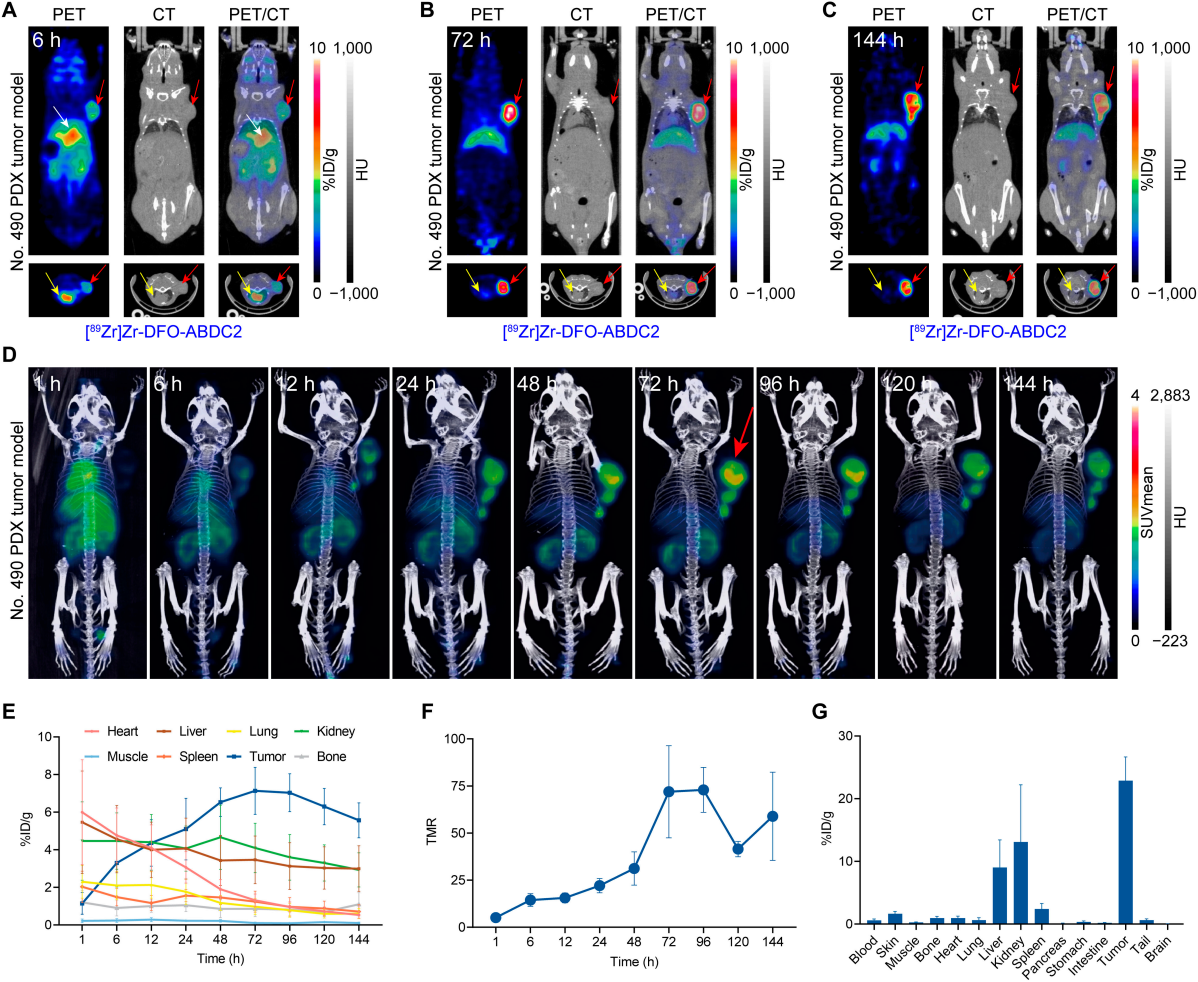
[^89^Zr]Zr-DFO-ABDC2 immunoPET imaging in No. 490 gastric cancer PDX models (4.10 ± 0.50 MBq, *n* = 3). Representative coronal (top panels) and axial (bottom panels) [^89^Zr]Zr-DFO-ABDC2 immunoPET/CT images at 6 h (A), 72 h (B), and 144 h (C). The tumors, hearts, and abdominal aortas were indicated by red arrows, yellow arrows, and white arrows, respectively. (D) The maximum intensity projection images fused with CT images of all the time points show the distribution patterns of [^89^Zr]Zr-DFO-ABDC2. (E) ROI data showing the uptake kinetics of [^89^Zr]Zr-DFO-ABDC2 at different time points. (F) Tumor-to-muscle ratio (TMR) analysis at different time points after injection of the tracer. (G) Ex vivo biodistribution data showing the detailed uptake and distribution of [^89^Zr]Zr-DFO-ABDC2.
